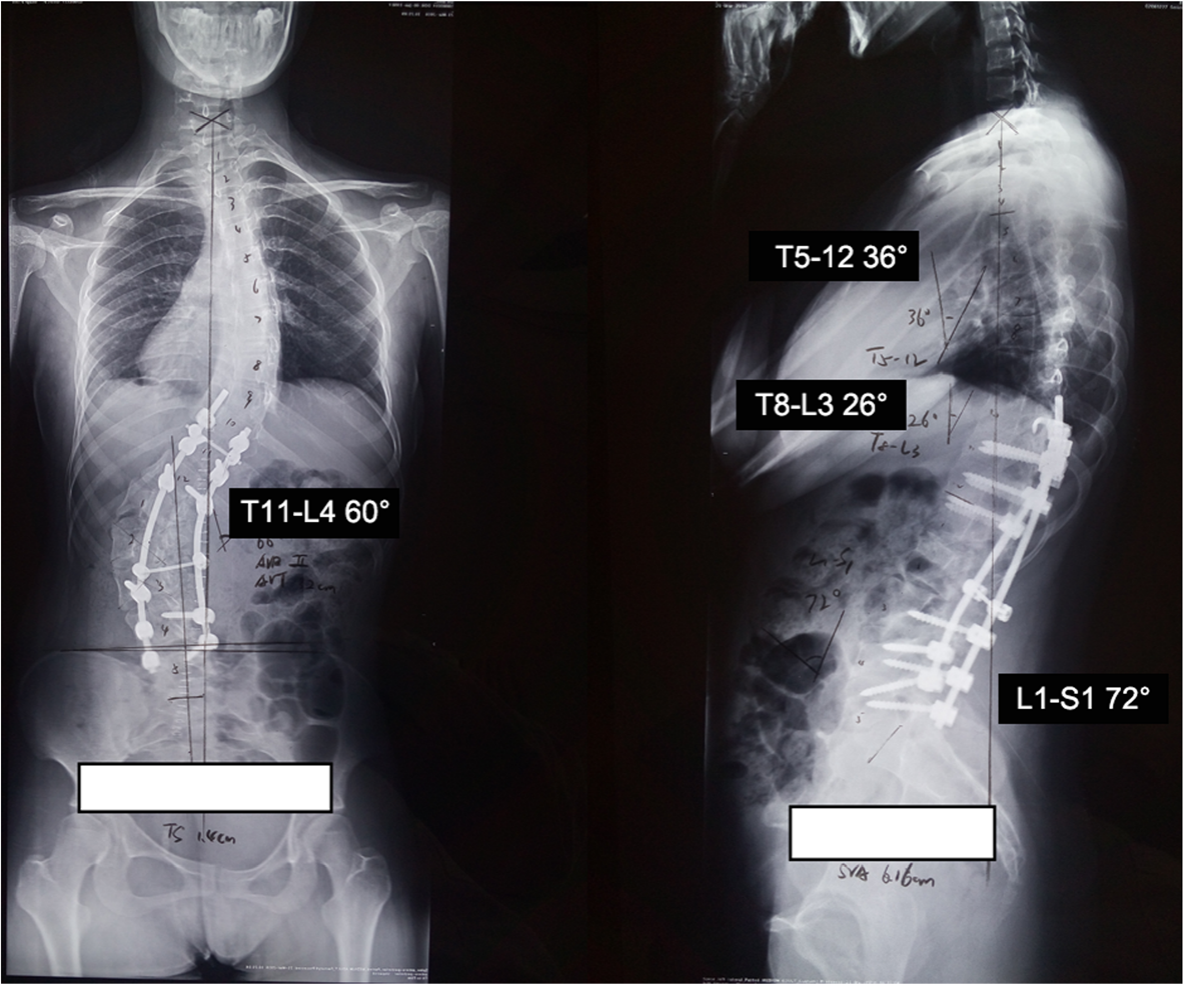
Post-operative standing anteroposterior and lateral radiographs
